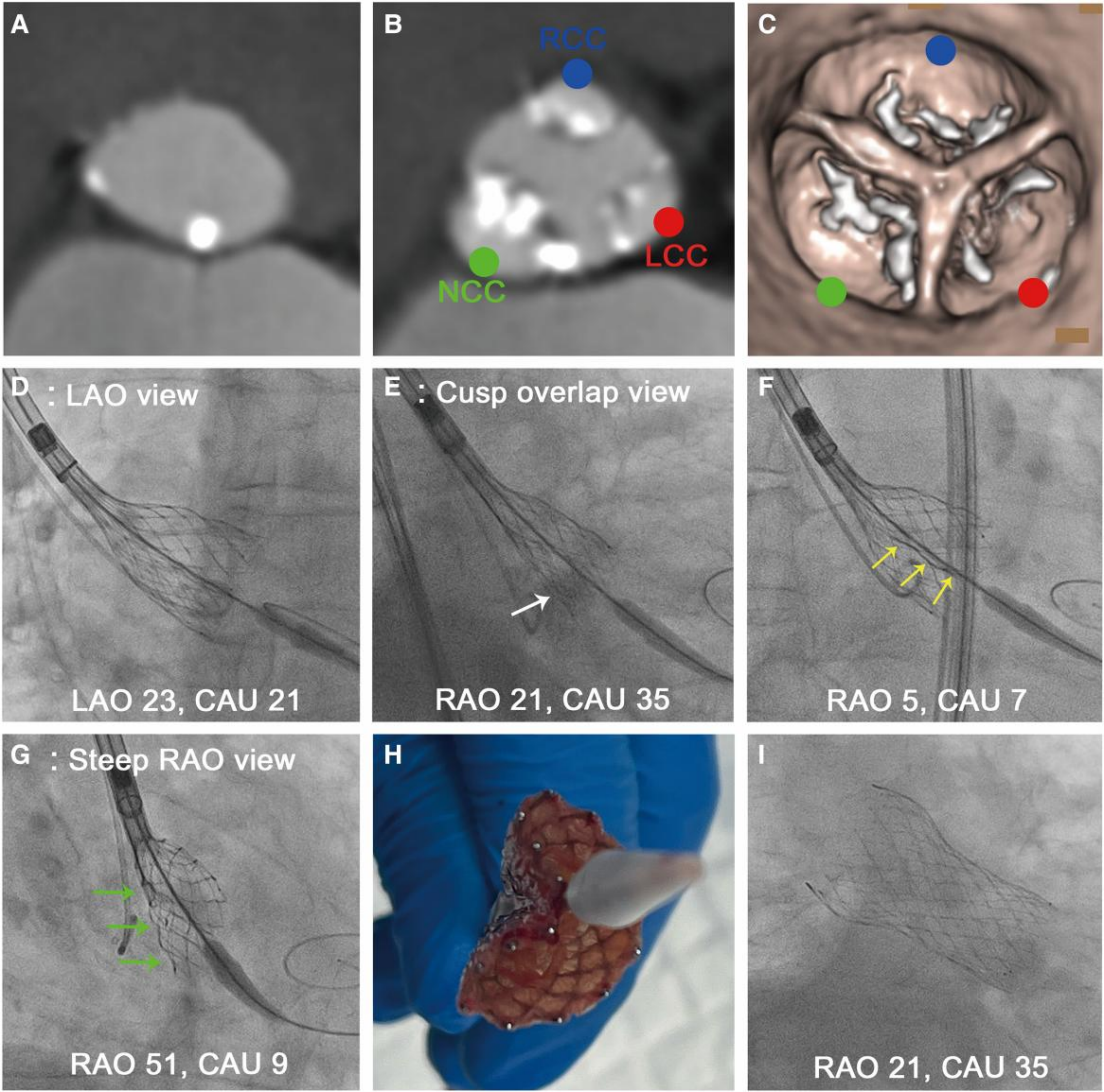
Transcatheter heart valve infolding diagnosed in the steep right anterior oblique view. (*A–C*) Baseline computed tomography imaging showing a heavily calcified aortic valve, especially in the non-coronary cusp. (*A*) Aortic annulus. (*B*) 6 mm above the annulus. (*C*) Volume-rendering image. (*D*) The transcatheter heart valve appeared to be well expanded in the left anterior oblique view. (*E*) In the cusp-overlap view, it appeared to be slightly under-expanded (white arrow). (*F*) Using rotational fluoroscopy, a vertical line along the valve frame was suspected (yellow arrows). (*G*) Significant stent distortion, particularly in the non-coronary cusp side, was first identified in the steep right anterior oblique view (green arrows). (*H*) Infolded transcatheter heart valve. (*I*) A new transcatheter heart valve was successfully implanted with full expansion. The blue circle represents the right-coronary cusp; the red circle represents the left-coronary cusp; and the green circle indicates the non-coronary cusp.
